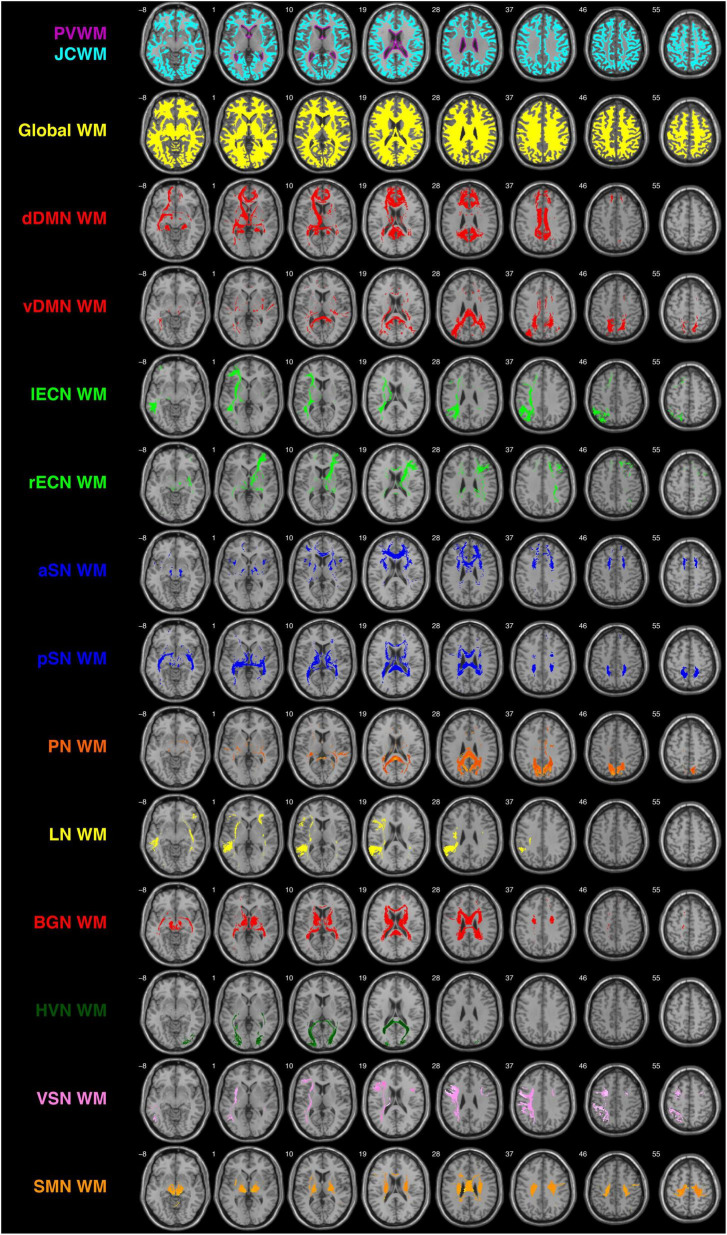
Depictions of the PVWM and JCWM masks (top row), global WM mask (second row), and each of the 12 functionally defined human white matter networks investigated in the current study (rows 3–14) overlaid on the single-subject T1-weighted ICBM152 template. dDMN, dorsal default mode network; vDMN, ventral default mode network; lECN, left executive control network; rECN, right executive control network; aSN, anterior salience network; pSN, posterior salience network; PN, precuneus network; LN, language network; BGN, basal ganglia network; HVN, higher visual network; VSN, visuospatial network; SMN, sensorimotor network.
